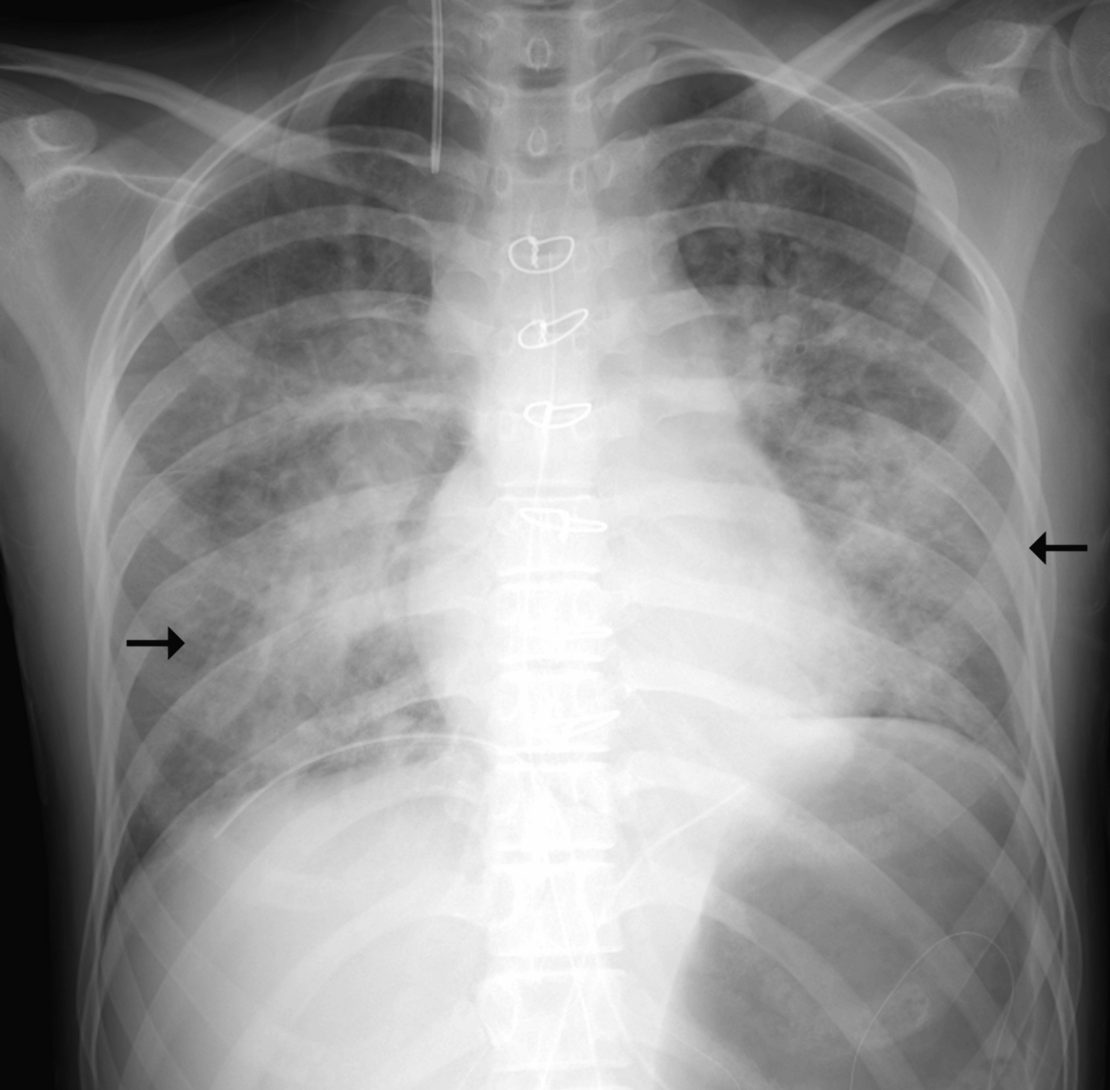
Chest radiograph obtained after aspiration showed diffuse bilateral consolidation (arrows)
